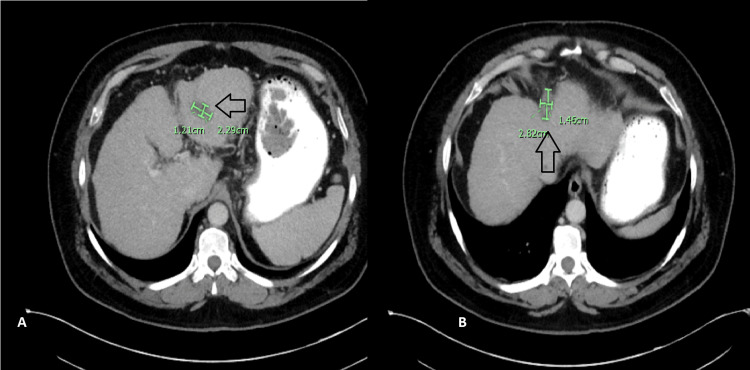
December 2021 CT scan of the abdomen A durable radiological response of the masses is seen (arrows in A and B)
